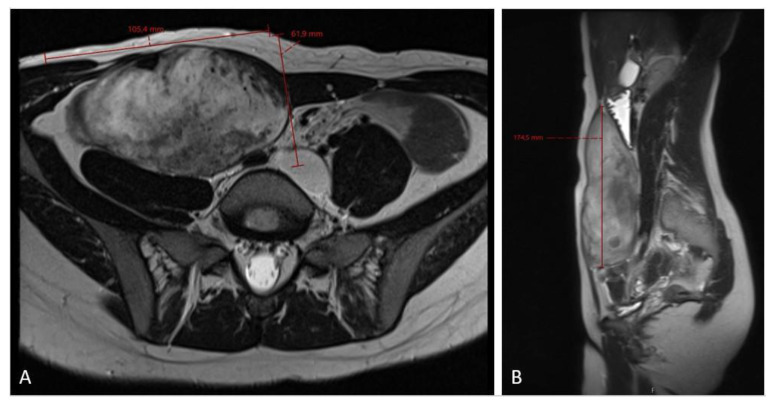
An MRI scan ((A)—axial plane; (B)—sagittal plane) at the 6-month follow-up checkup showing tumor growth progression, sized 10.5 × 6.2 × 17.5 cm in craniocaudal diameter, located in the musculus rectus abdominis on the right side.
